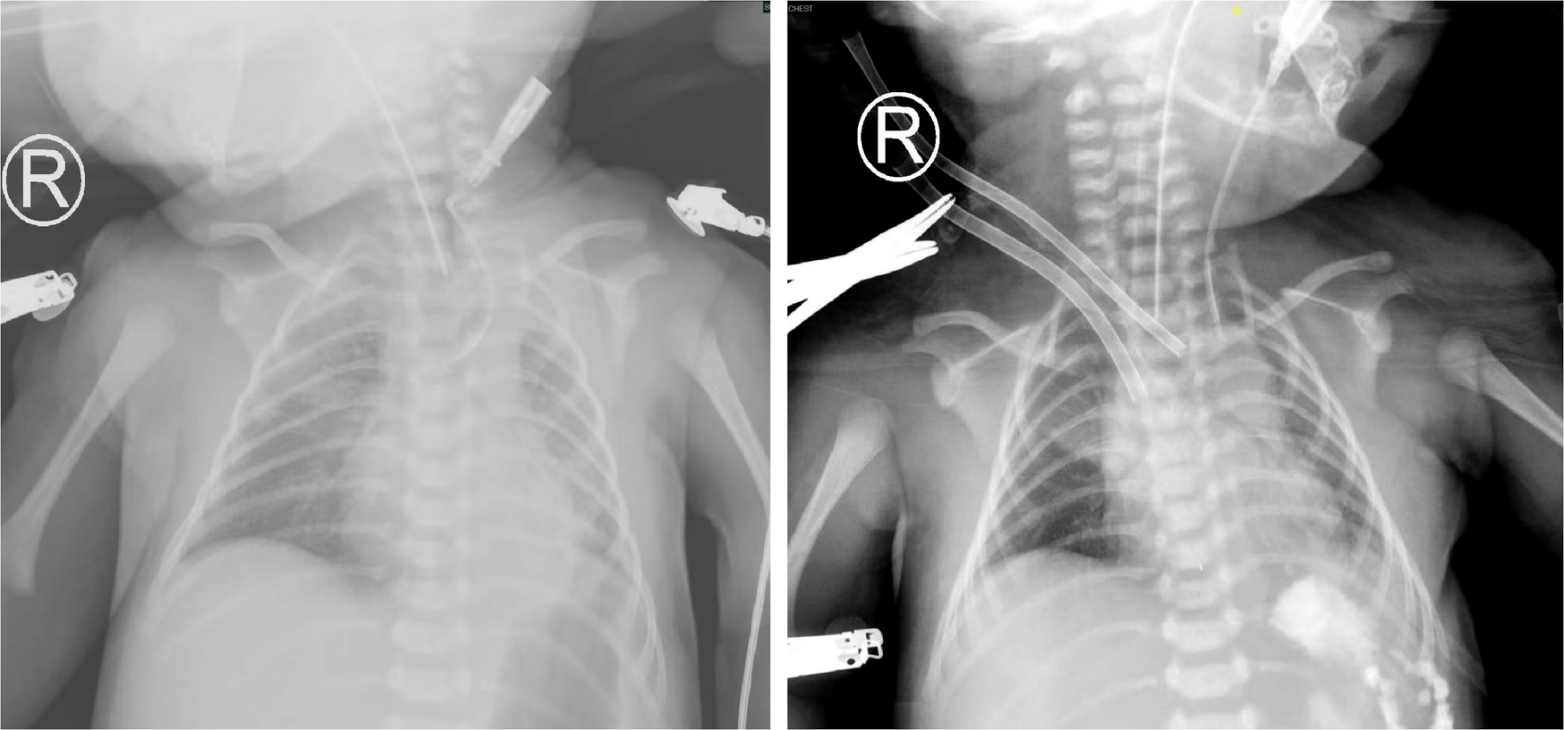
x-ray before ECMO treatment and during ECMO treatment in case 3.
